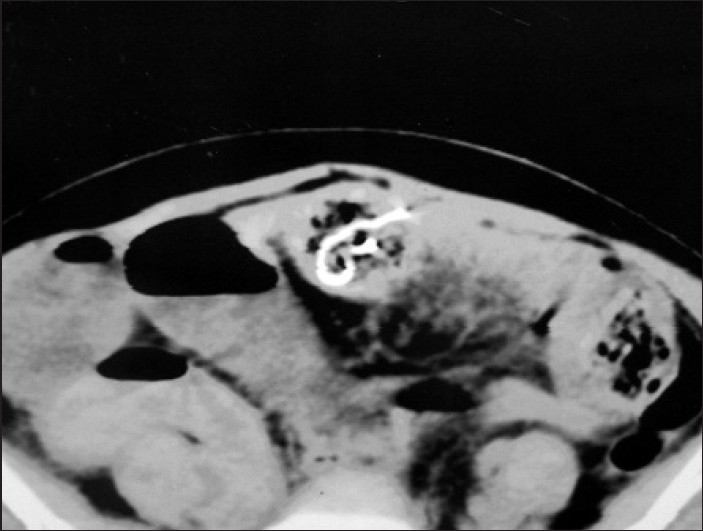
CT scan showing marker of mop
